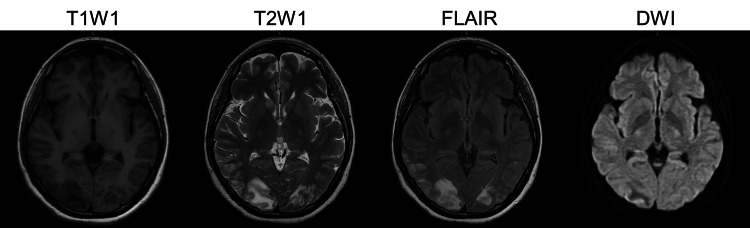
MRI of maternal brain (axial) showing posterior reversible encephalopathy syndrome in a postpartum patient with eclampsia and SLE. T2-weighted, FLAIR, and DWI sequences reveal characteristic hyperintense lesions in the occipital region. T1WI: T1 weighted image; T2WI: T2 weighted image; FLAIR: fluid-attenuated inversion recovery; DWI: diffusion-weighted image.
